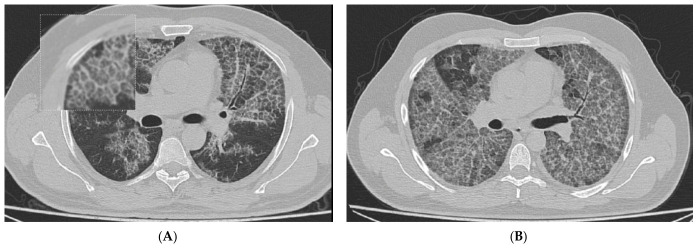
Representative thoracic HRCT images of a PAP patient from the study cohort. (A,B) Axial sections show extensive, bilateral ground-glass opacities superimposed on a network of thickened septa. This “crazy-paving” pattern visually represents the heavy intra-alveolar lipoproteinaceous accumulation measured in our quantitative analysis.
